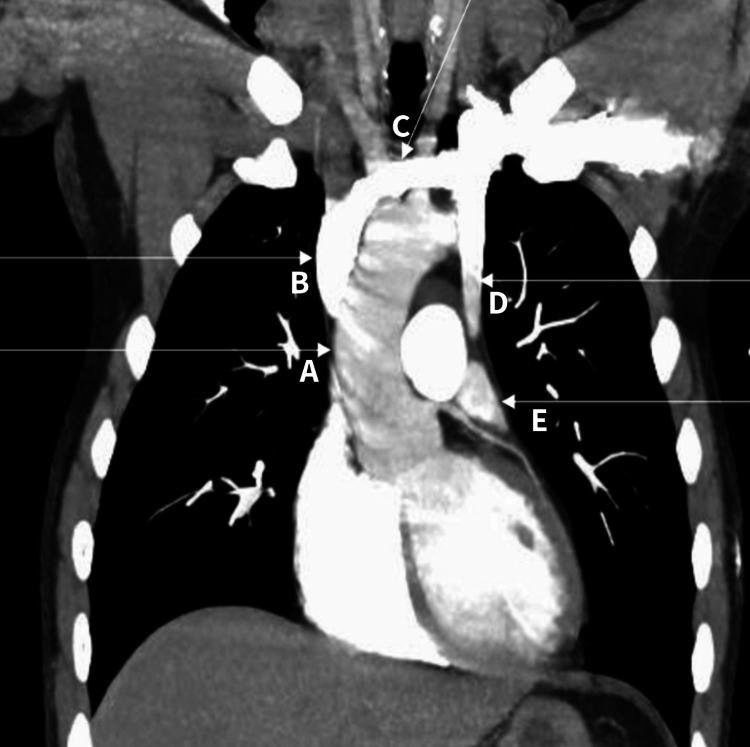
CT pulmonary angiography shows an anomalous persistent left superior vena cava. CT pulmonary angiography revealed a normal superior vena cava (B) and aorta (A). An anomalous persistent left superior vena cava (D) arising from the left innominate vein (C) and draining into the left atrium (E) is evident.
